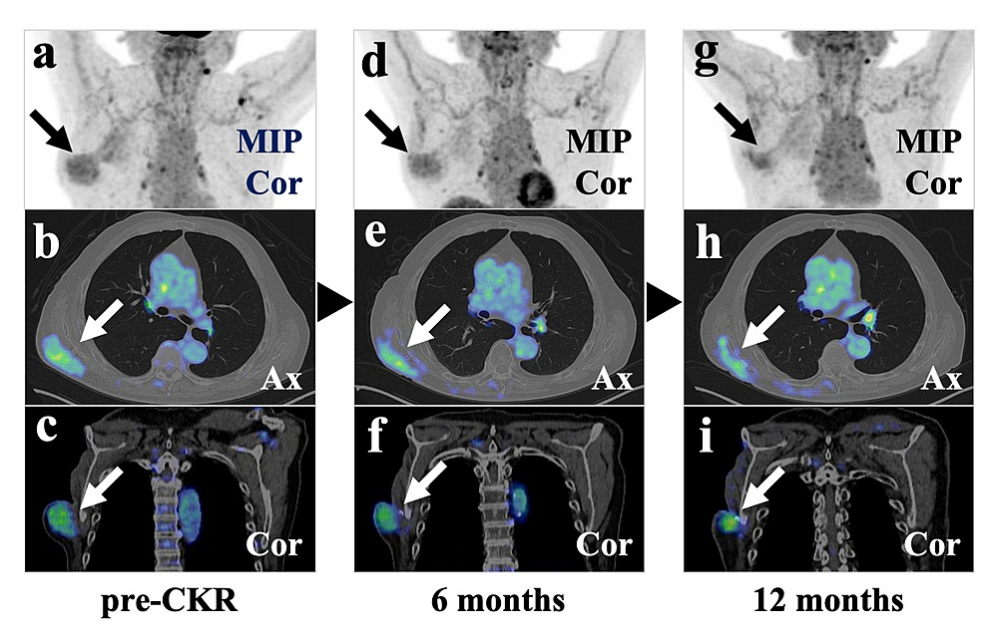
Time course of FDG-PET/CT (a), (b), and (c). A scapula side lesion with relatively high FDG uptake in the right posterior chest wall before CKR (black and white arrows)
